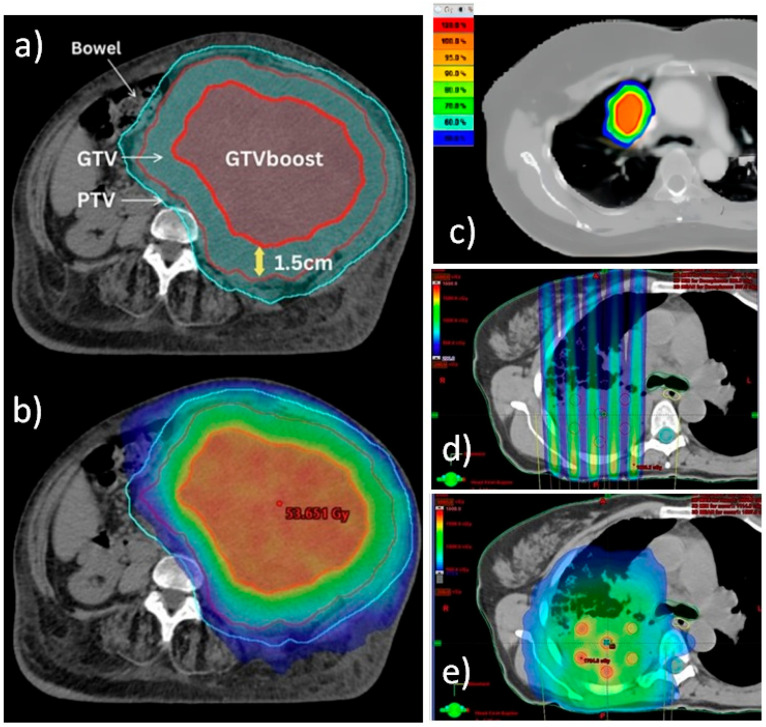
Comparison of dose distribution patterns of PART (a,b), SBRT (c), SFRT (d), and LRT (e) techniques. (a,b) Definition of target volumes and dose wash isodoses for PART planning for a bulky unresectable sarcoma. Courtesy of reference [9]. (a) Choice of authors for targets delineation. Diagnostic CT/MRI or PET/CT were fused with CT planning and the GTV was contoured. Next, a 1.5 cm isotropic contraction from the GTV was applied to form the GTV boost inside the GTV. Then, a 1 cm isotropic expansion from GTV was applied to generate the PTV. (b) Relative dose distribution prescribing 20 Gy to PTV (blue isodose) and 50 Gy to GTV boost (red isodose). (c) Typical dose distribution for an SBRT treatment of a lung lesion. Courtesy of reference [33]. Isodoses range from 50% (blue) to 100% (red) of dose prescription. (d,e) Irradiation of a bulky lung tumor. Courtesy of reference [27]. (d) Typical non-uniform radiation dose distribution of SFRT planning across the target volume, using grids to create spatially fractionated dose patterns in the form of high-dose and low-dose regions. Note that for deep-seated bulky tumors, SFRT faces the problem of high doses falling into normal tissue. (e) Typical dose distribution of LRT irradiation, where the creation of multiple localized high-dose small spheres called vertices with a certain degree of separation within the tumor volume is clearly visible. This technique also allows the dose level to be kept lower in the periphery of the tumor to avoid related toxicity.
